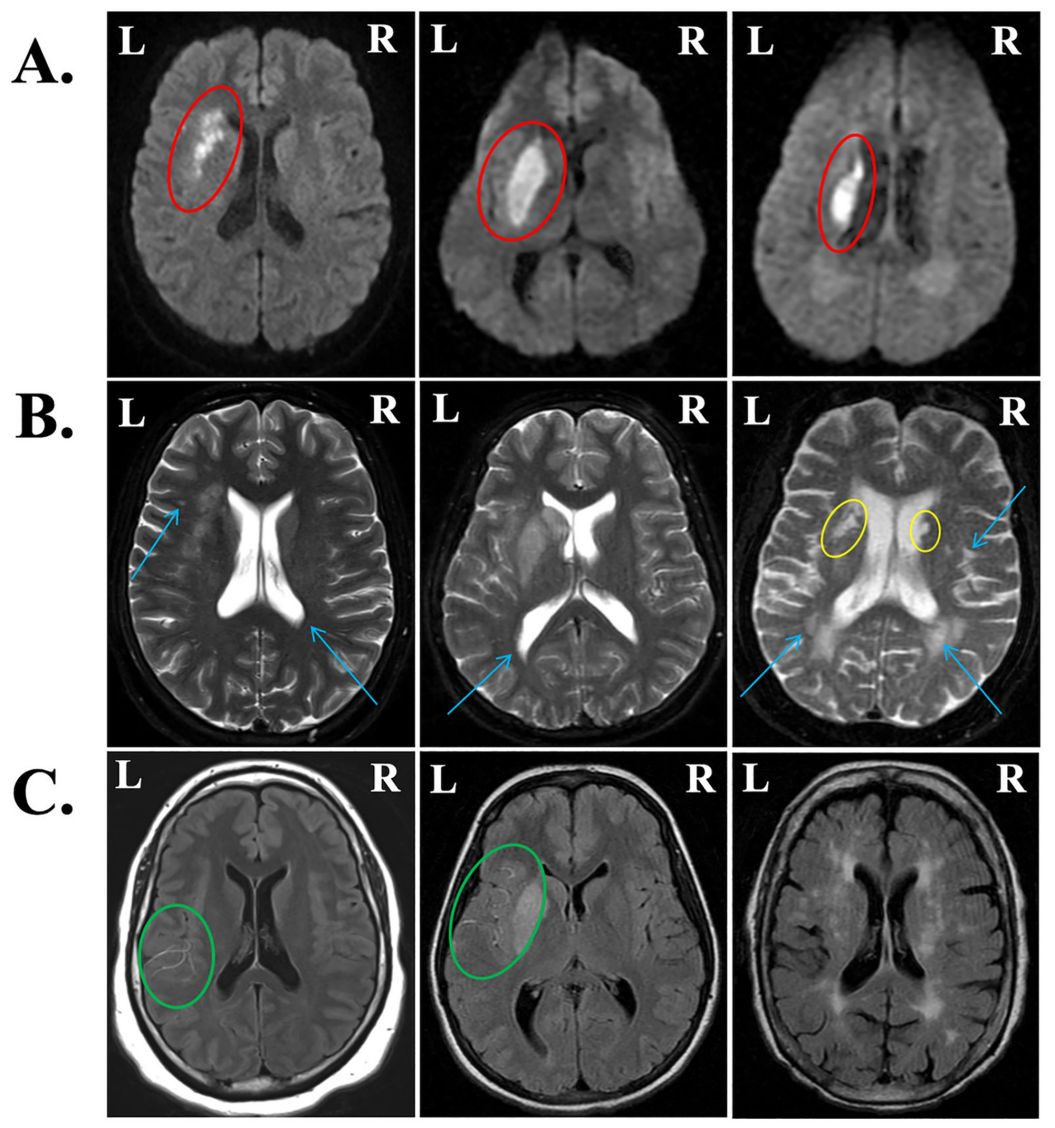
Representative images from patients with varying acute and chronic infarct volumes, leukoaraiosis, and hypoperfusion. (A) Diffusion-weighted images (DWI) display acute lesions (red ovals). (B) T2-weighted scans were used to visually score leukoaraiosis on the Fazekas scale (blue arrows) from 0 to 3 and visualize chronic infarcts where present (yellow ovals). From left to right, periventricular hyperintensities (PVH) were scored as 1, 1, and 3; deep white matter hyperintensities (DWMH) were scored as 1, 0, and 2. (C) FLAIR hyperintense vessels (FHV) are indicative of hypoperfusion in areas surrounding infarcted tissue (green ovals). From left to right, FHV scores were 5, 7, and 0 out of 12.
